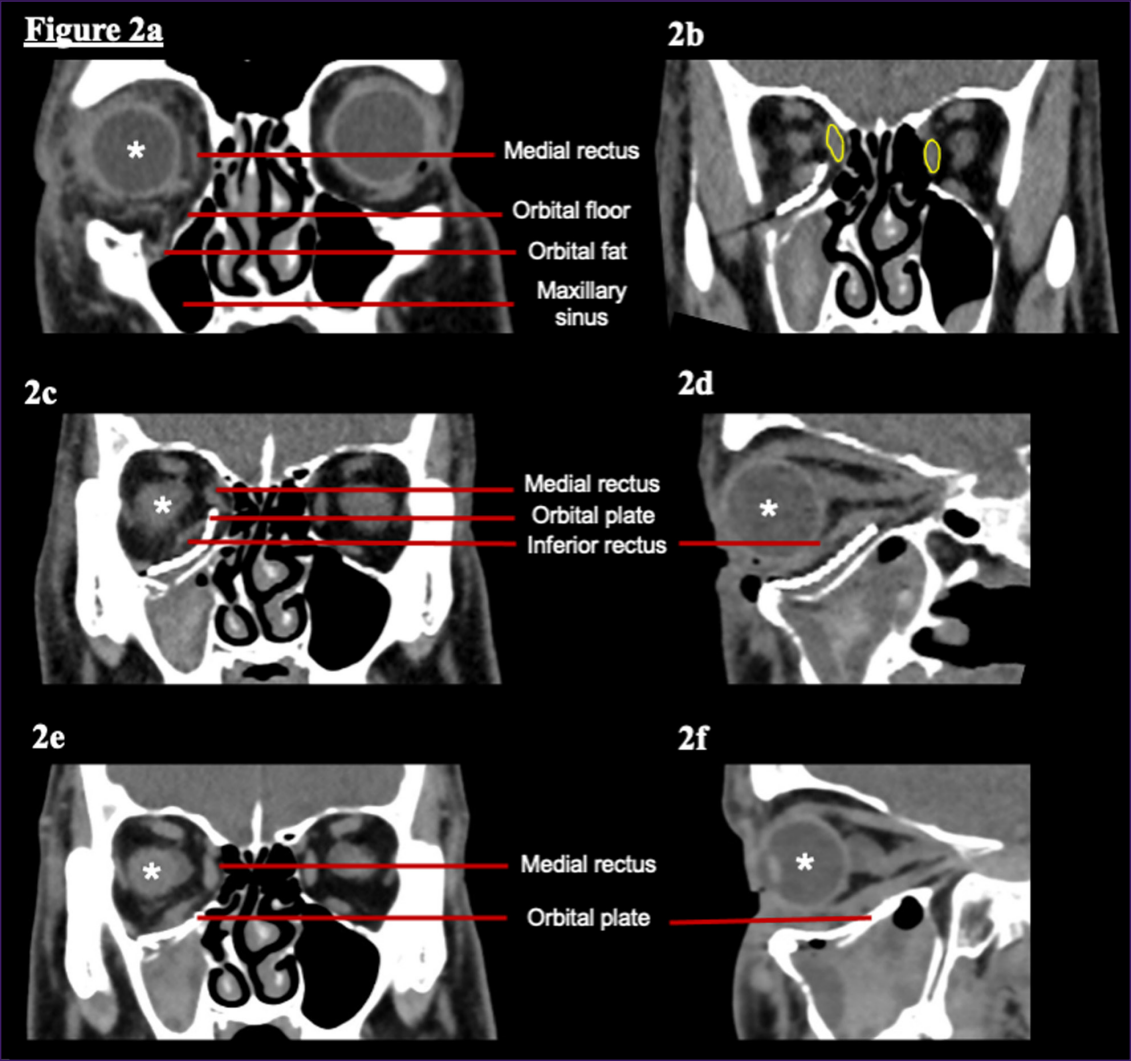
CT scan of orbit using soft tissue windows. 2a Orbital floor fracture following initial injury. 2b. Coronal section following orbital floor repair. The bodies of the left and right medial recti have been highlighted to demonstrate their asymmetric positioning. The right medial rectus is clearly elevated in comparison to the contralateral side. 2c. Coronal section of the orbit shows the medial rectus tendon in close proximity to and distorted by the orbital plate. Anatomical structures are labelled, * indicates the globe. 2d. Sagittal section shows posterior extension of orbital plate. 2e. Coronal section following orbital plate exchange shows right medial rectus now symmetrical with left. 2f. Sagittal section following orbital plate exchange shows less posterior extension of orbital plate.
